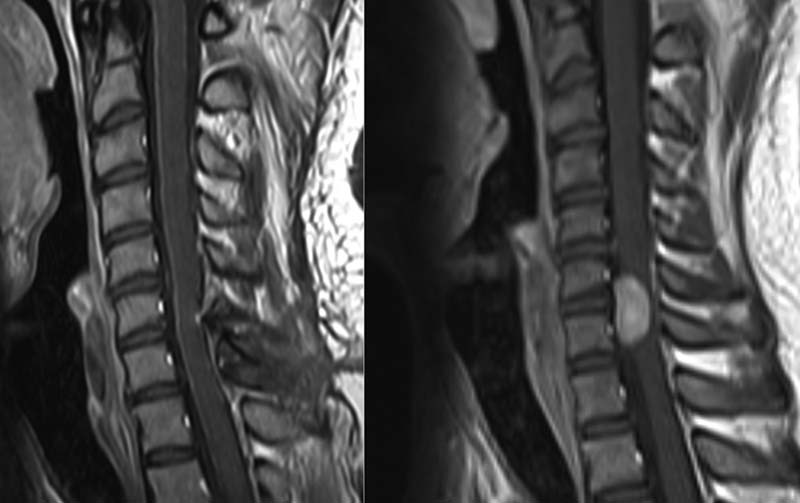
Sagittal T1-weighted magnetic resonance imaging of the first case preoperatively (right) showing a bright signal denoting an intradural extramedullary tumor and 1 month postoperatively (left) showing complete removal.
